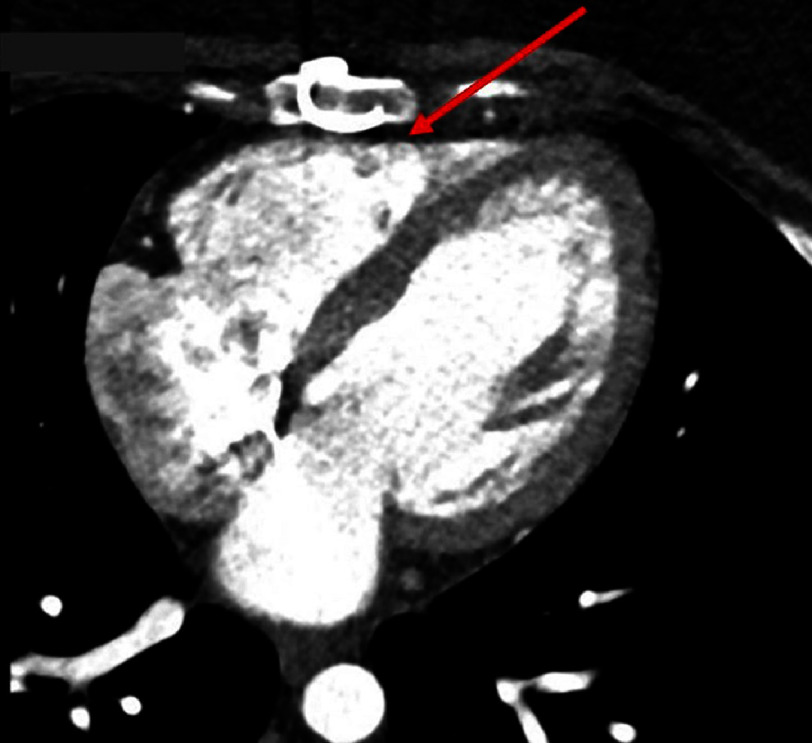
Axial image of computed tomography (CT) scan of chest with contrast demonstrates right ventricular wall near the posterior table of the sternum.
